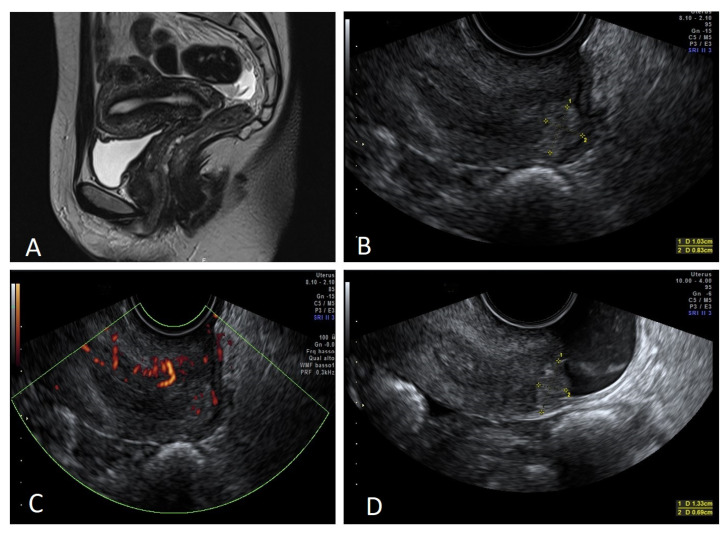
Small tumor after conization. (A) Not detectable disease by MRI. (B) Detectable disease by TVUS, measuring 10.3 × 8.3 mm. (C) Lesion vascularization by power Doppler. (D) Disease detectable by VGS, measuring 13.3 × 6.9 mm.
